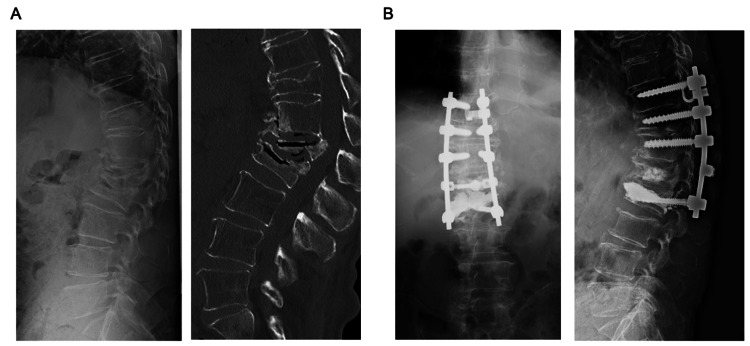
Future prospects: clinical case of a 73-year-old male Chief complaint: Inability to walk because of back and leg pain. (A) Preoperative imaging (X-ray and computed tomography): The L1 and L2 vertebral bodies exhibited pseudarthrosis with the presence of intravertebral vacuum phenomena. Bony fusion was observed in more than four vertebrae proximal to T12, confirming the findings of diffuse idiopathic skeletal hyperostosis. (B) Postoperative imaging (X-ray): Balloon kyphoplasty was performed at the L2 vertebral body, and cement-catching screws were inserted as a caudal anchor. No screw pullout was observed at six months postoperatively.
